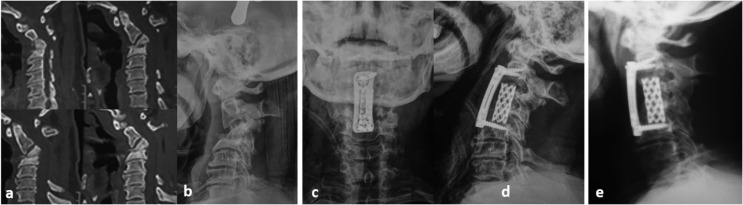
SD C3–4 in a 62-year-old male. (a) Preoperative CT scan showing C3–4 SD with significant local kyphosis. (b) Preoperative lateral X-rays after traction for 2 weeks. (c and d) Postoperative X-rays anteroposterior and lateral views showing corpectomy of C3 and C2–4 fusion using titanium cage and cervical locked H-shaped plate. (e) Six months follow-up lateral X-rays showing solid fusion.
